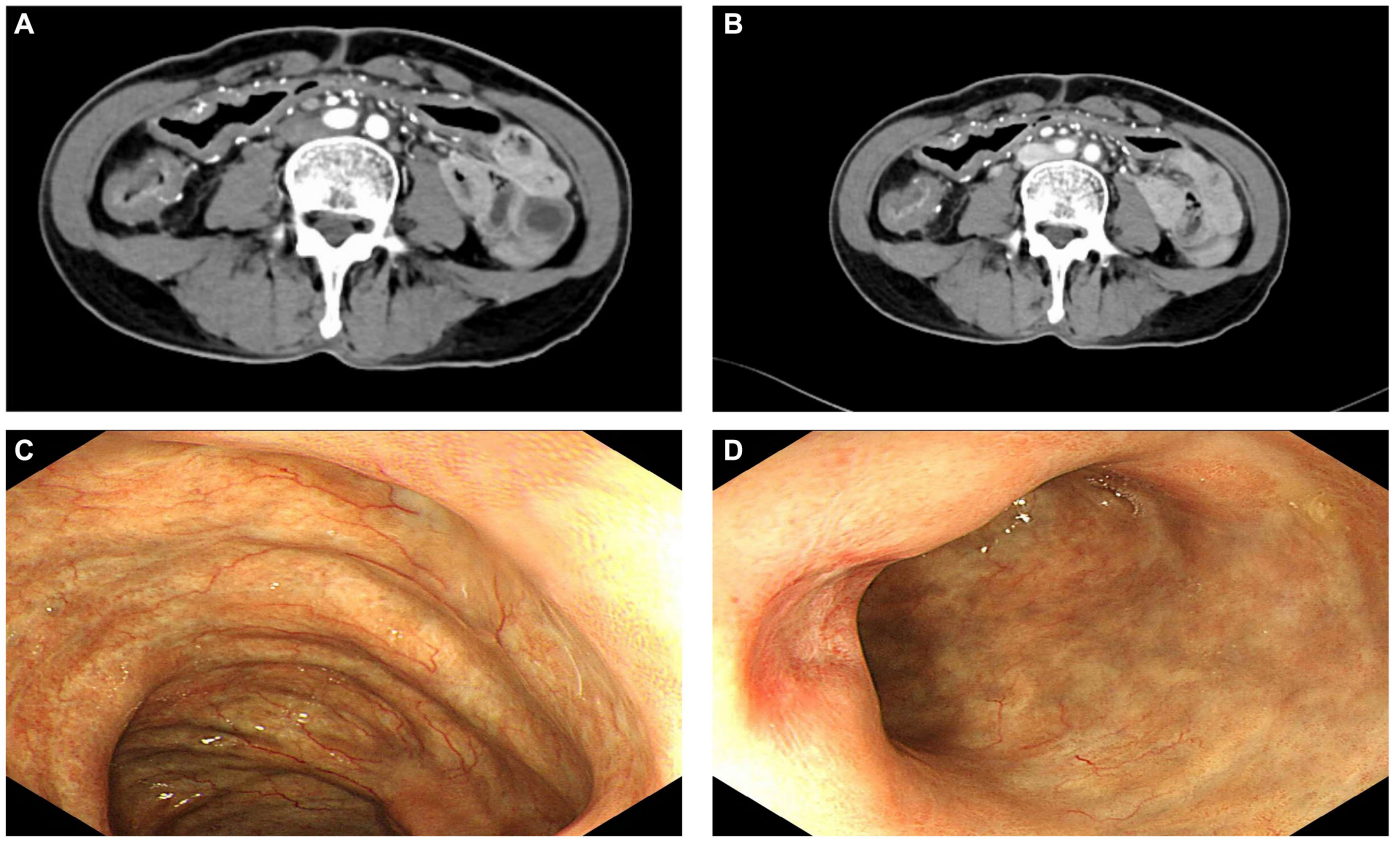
Preoperative abdominal computed tomography (A,B) and colonoscopy results (C,D).
